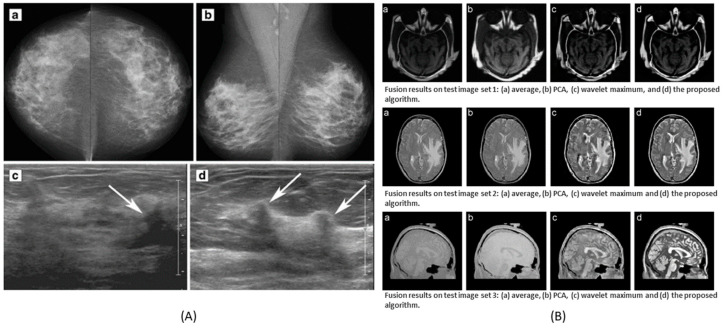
Data fusion as a result of different types of images. (A) Ultrasound and mammogram image fusion to detect breast cancer. (B) CT and MRI image fusion to provide high spatial quality of anatomical information and functional details of the diseases. (A) is reprinted with permissions from reference [36]. (B) is adapted with permissions from reference [37], 2008, Elsevier.
